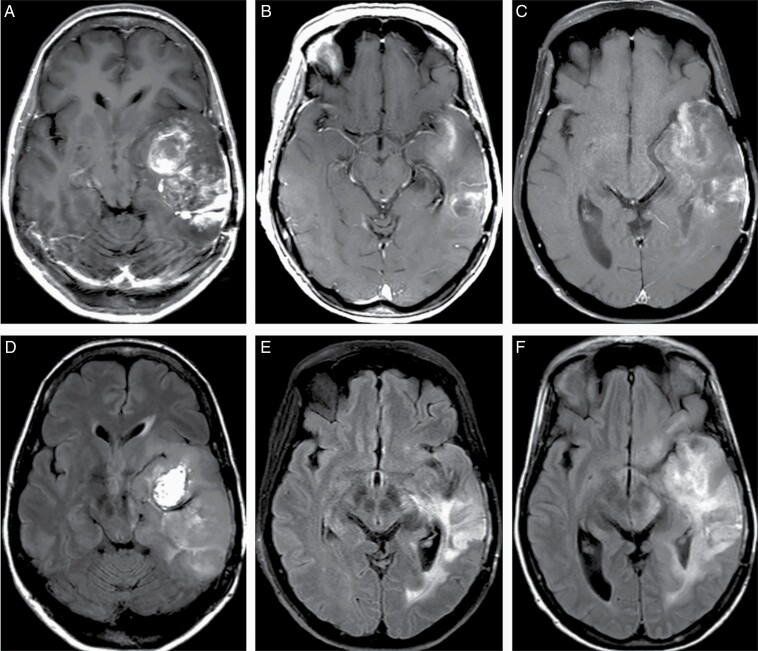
Pseudoresponse following bevacizumab therapy in a 15 year old male patient with recurrent high grade glioma with histone H3.3 G34 mutation in the left temporal lobe. Extensive heterogenous enhancement (A, post-contrast T1-weighted image) and edema with mass effects (D, FLAIR image) have been significantly reduced 3 weeks after the start of bevacizumab therapy (B, E, post-contrast T1-weighted image and FLAIR image, respectively); however, there is evidence of worsening of enhancement (C, motion degraded post-contrast T1-weighted image) and infiltrative tumor component (F, FLAIR image), on a follow-up MRI obtained 8 weeks after the start of bevacizumab therapy.
